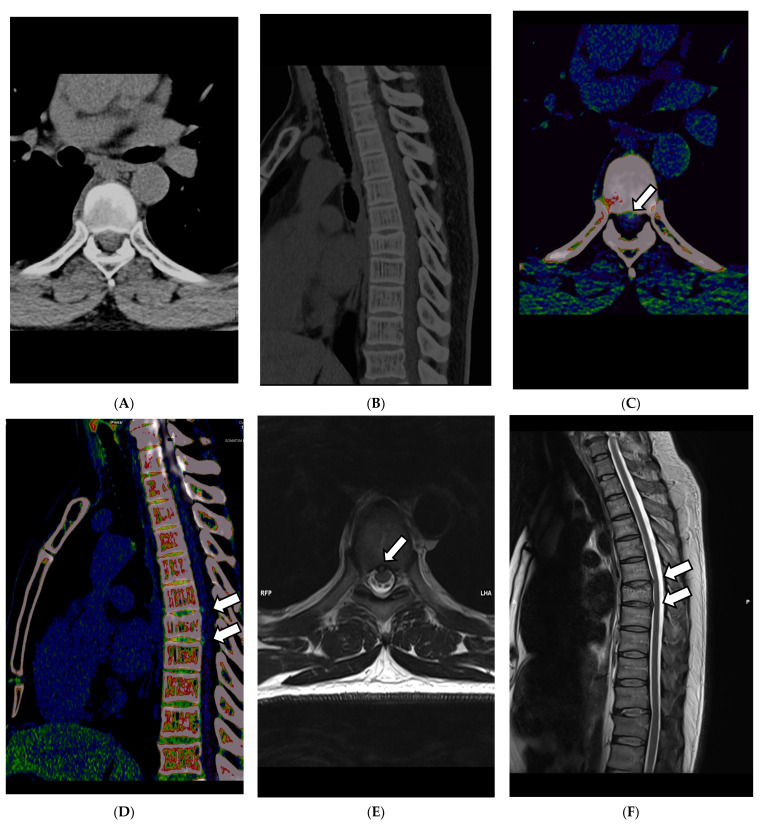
DECT scan of the thoracal spine in a 61-year-old female patient that presented herself with focal pain at the upper back in the clinical examination after slipping and falling on her back. The clinicians suspected a possible vertebral fracture. Thus, a CT scan without a contrast agent was scheduled. During the reading of the images, fractures were ruled out quickly. In the VMI 120 kV soft tissue kernel CT reconstruction, as shown in (A) axial and (B) in sagittal orientation, disk herniation was hardly visible. Application of color-coded VNCa DECT reconstructions finally revealed dorsal median disc herniations (indicated by the white arrows in (D,F)) in segments T 6/7 and T 7/8, as seen in (C) in axial orientation and (D) in sagittal orientation. The finding was later verified in a 1.5 Tesla MRI examination, where the disc herniation could be correlated to the CT findings, as seen in the axial T2-WI sequence (E) and the sagittal T2-WI sequence (F).
